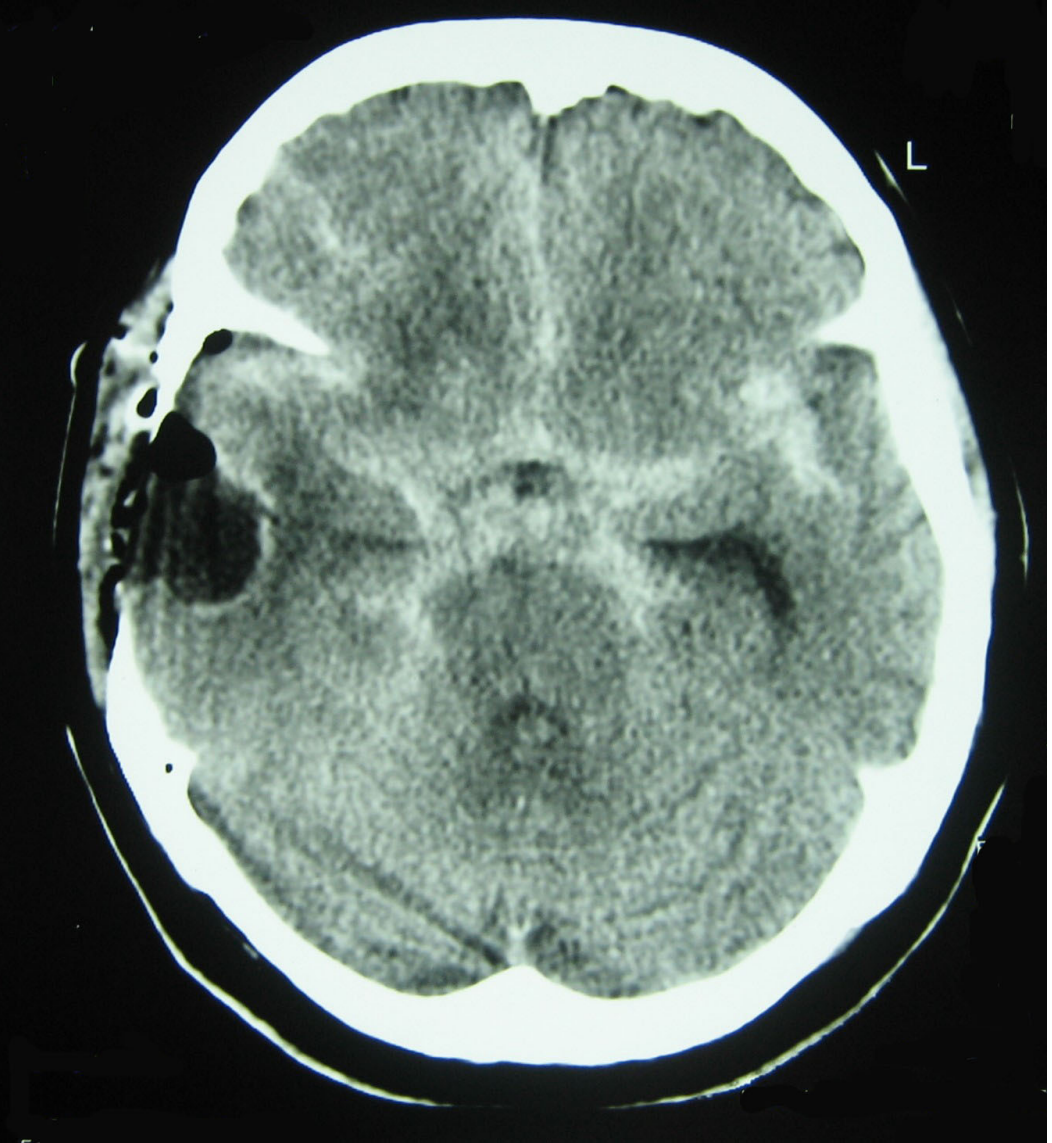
Post operative CT image shows diffuse subarachnoid haemorrhage in all basal cisterns, bilateral sylvian fissure and interhemispheric fissure. On the right side postoperative site of tumour removal is seen.
